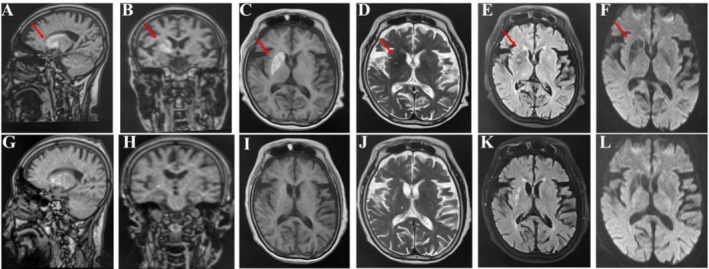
Serial brain MRI of the right basal ganglia. The top row (A–F) displays images from the initial presentation on August 5, 2020, while the bottom row (G–L) shows follow‐up imaging from May 25, 2021. The initial scan reveals a lesion in the right putamen (red arrows) characterized by distinct hyperintensity on T1‐weighted imaging (C) and corresponding hypointensity on T2‐weighted (D), T2‐FLAIR (E), and Diffusion‐Weighted Imaging (DWI) (F) sequences. The follow‐up imaging (G–L) demonstrates a near‐complete resolution of these signal abnormalities, particularly the T1 hyperintensity (I).
